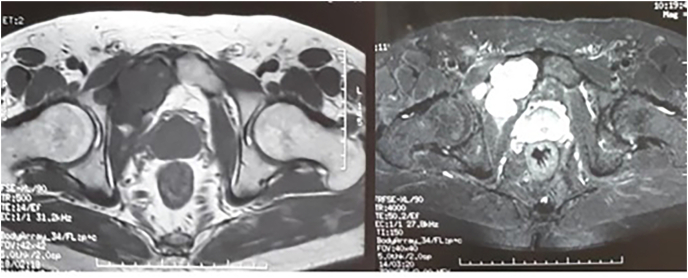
MRI showing a lytic lesion of right pecten pubis with a low intensity in T1, and heterogeneous characteristics in T2-weighted sequences.
